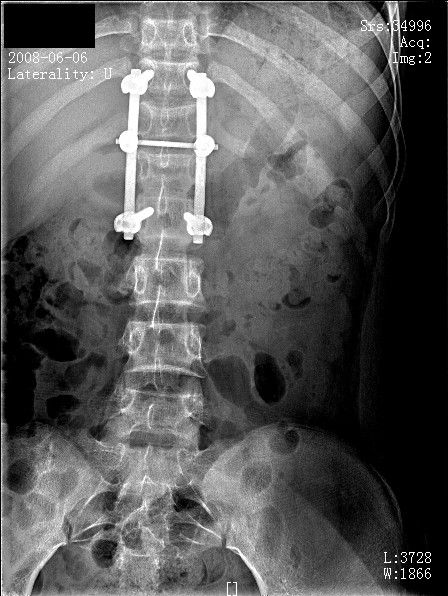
Post-operative radiograph (anteroposterior view) with posterior spinal fusion performed.
